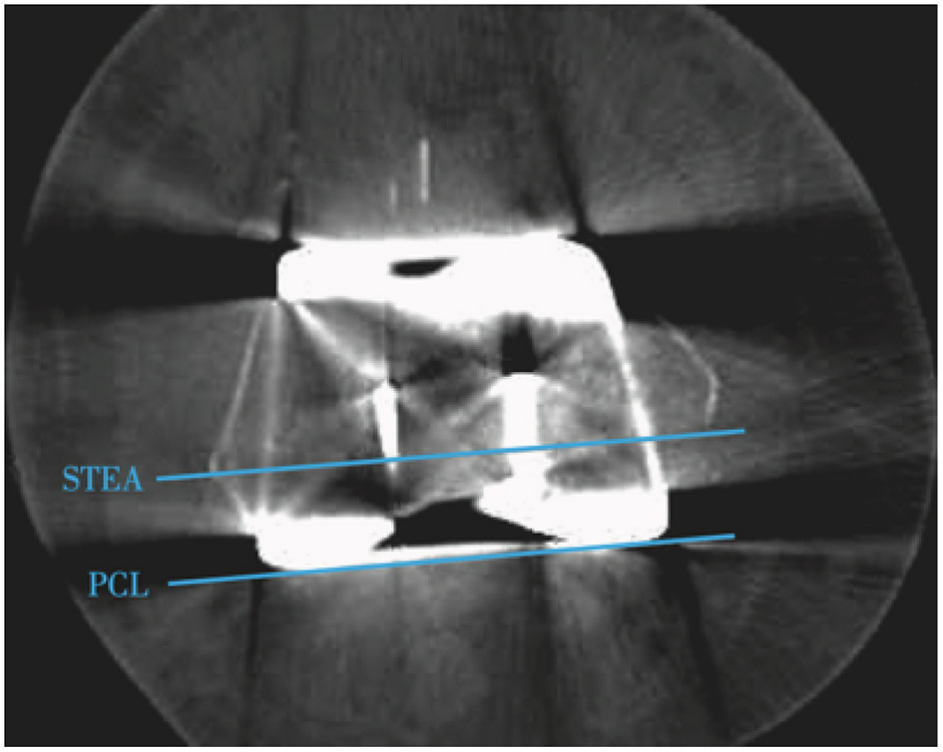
Postoperative CT of knee. The STEA (surgical transepicondylar axis) represents the axis of rotation of the original femur of the knee, while the PCL (posterior condyle line) of the prosthesis can be regarded as the axis of rotation of the prosthesis. The femoral prothesis rotation angle reflects the difference of the femoral prosthesis rotation axis with the surgical transepicondylar axis.
